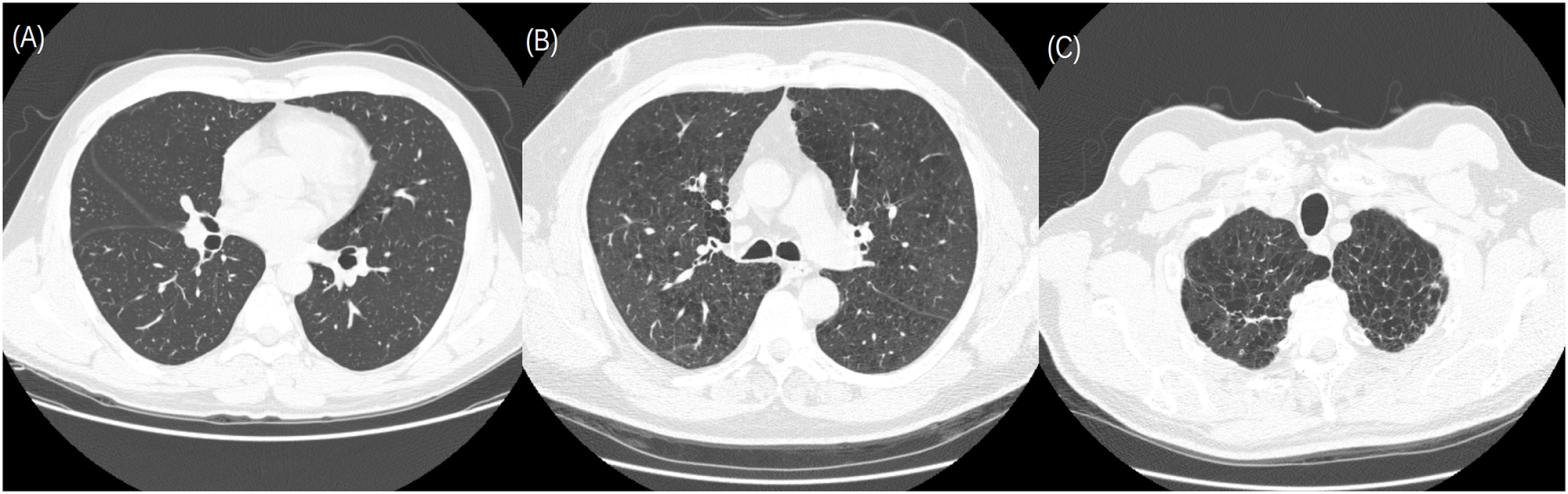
Representative CT images in the database. (A) visual score = 0 (no emphysema); (B) visual score = 3 (moderate); (C) visual score = 5 (very severe). The CT images were displayed with a lung window setting of 1600 HU window width and −550 HU window level.
